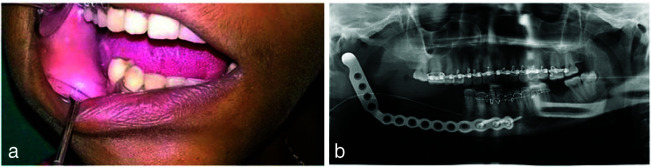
a: Follow up clinical picture of the patient after 6 months, b: Post operative radiograph of the patient
